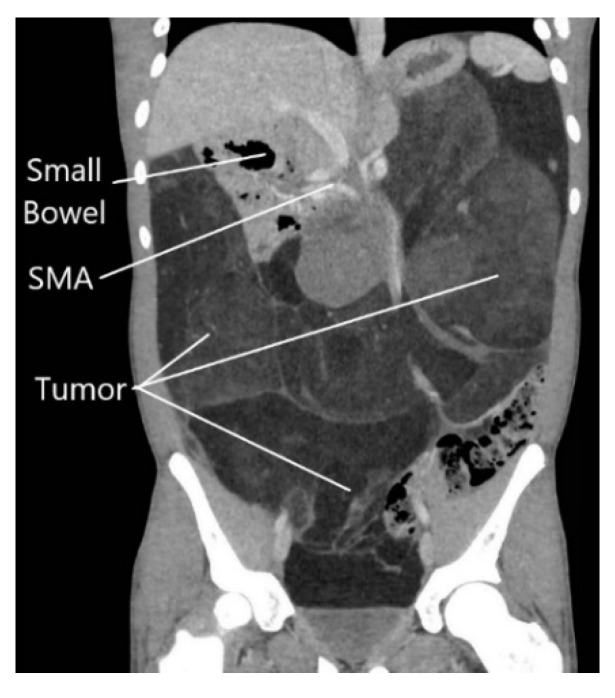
Abdominal computed tomography scan showing massive retroperitoneal liposarcoma, with displacement of the small intestine to the right upper quadrant.
